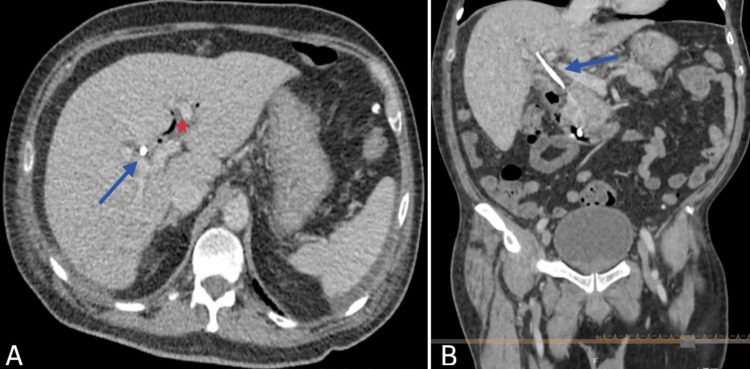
Non-contrast abdominopelvic CT scan. (A) Axial view: The gallbladder is not visualized. The blue arrow indicates hyperdense material within the common bile duct, corresponding to biliary diversion surgical material. The asterisk denotes pneumobilia. (B) Coronal reconstruction: The gallbladder remains absent. The blue arrow highlights a linear hyperdense structure within the bile duct lumen, extending distally toward the second portion of the duodenum. No associated fluid collections are observed.
